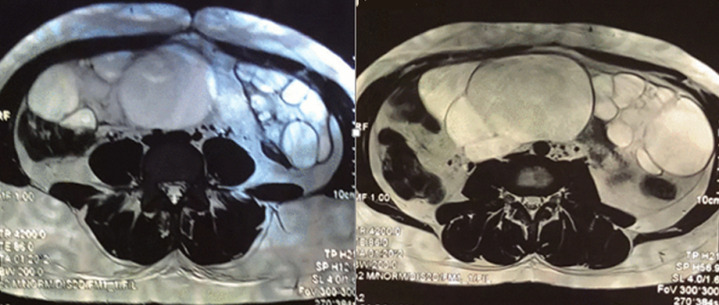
T2-weighted MRI image, axial plane through pelvis showing bilateral polycystic ovaries
